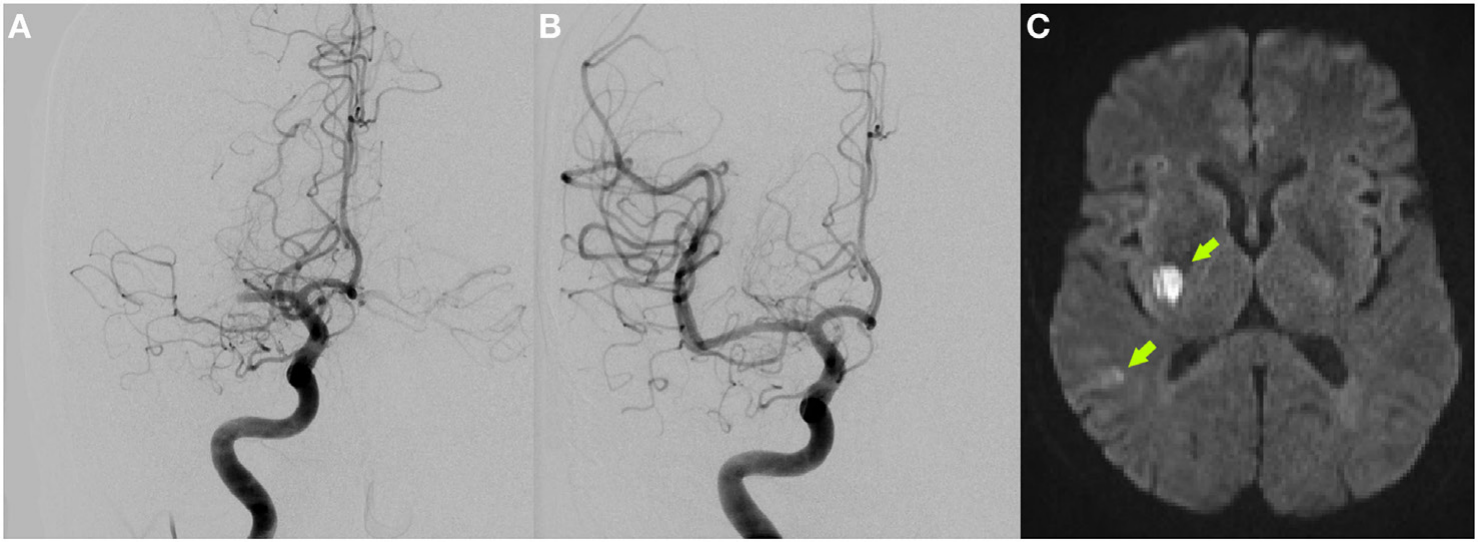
Initial digital subtraction angiography showing right proximal middle cerebral artery occlusion (A) and complete vessel recanalization after successful mechanical thrombectomy (B). DWI-MRI 4 days after stroke depicts small ischemic infarcts in the right posterior basal ganglia and temporal cortex [arrows (C)].
